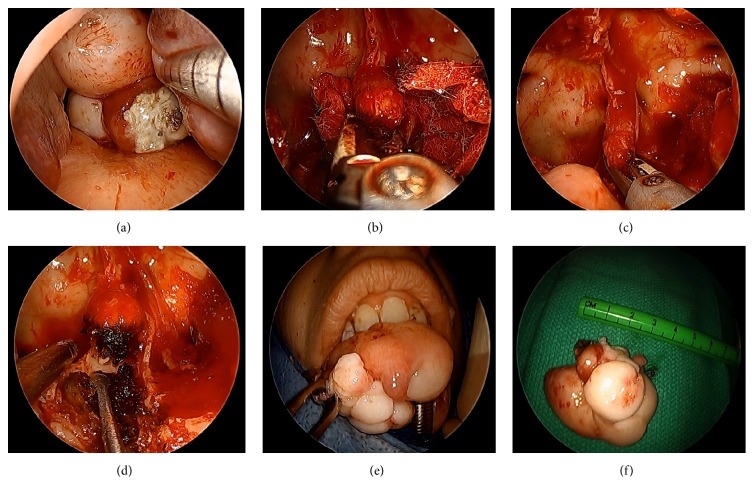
Surgical resection of skull base teratoma with ultrasonic bipolar diathermy. Endonasal view of the pedunculated skull base teratoma obstructing the posterior nasopharynx, with metal suction tip retracting the left inferior turbinate (a). Following sphenoidotomies, the tumor pedicle is approached (b), clamped in a full-thickness manner (c), and completely transected (d) using the vice-clamp tip and combined ultrasonic/bipolar platform energies. Teratoma passed into the oral cavity for transoral en bloc tumor removal (e), with lesion measuring approximately 4.0 cm in diameter (f).
